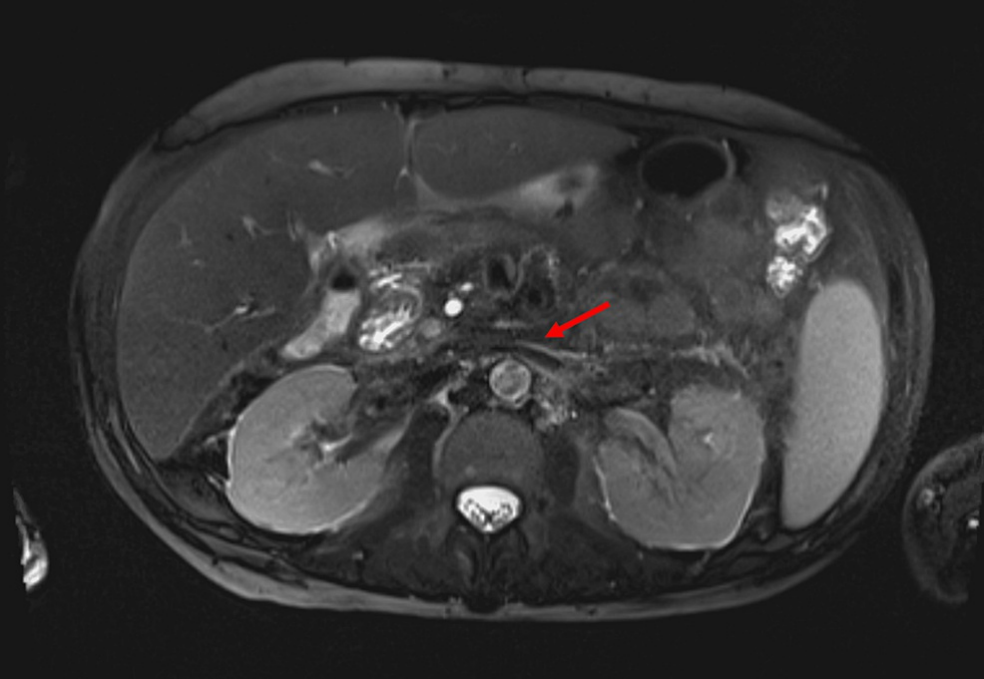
Magnetic resonance imaging showing retroperitoneal fibrosis (red arrow).
